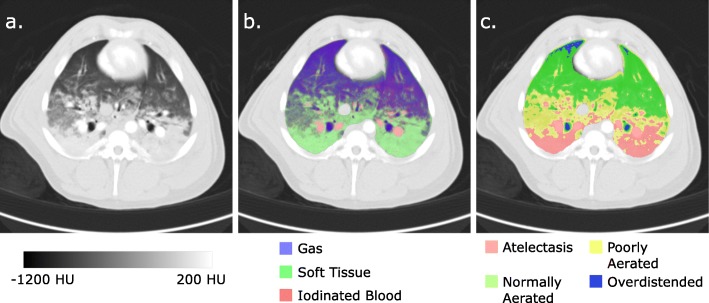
Example CT images of the juxtadiaphragmatic slice used in this study. a 70%:30% merge of the two dual-energy CT images using standard lung windows. b Results of segmentation to exclude extrapulmonary structures and dual-energy three material differentiation demonstrating gas, soft tissue and iodinated blood volume fractions. c Segmentation to identify regions of overdistension (gas volume fraction > 90%), normal aeration (gas volume fraction 50–90%), poor aeration (gas volume fraction 10–50%) and atelectasis (gas volume fraction < 10%). *HU* Hounsfield units
